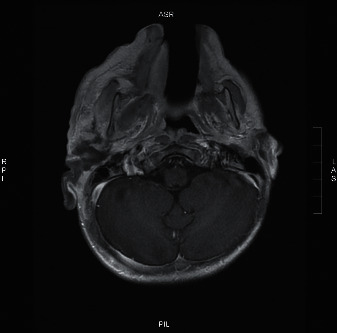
T1 MRI with gadolinium contrast showing significant inflammatory changes in the right ear canal, periauricular region.
